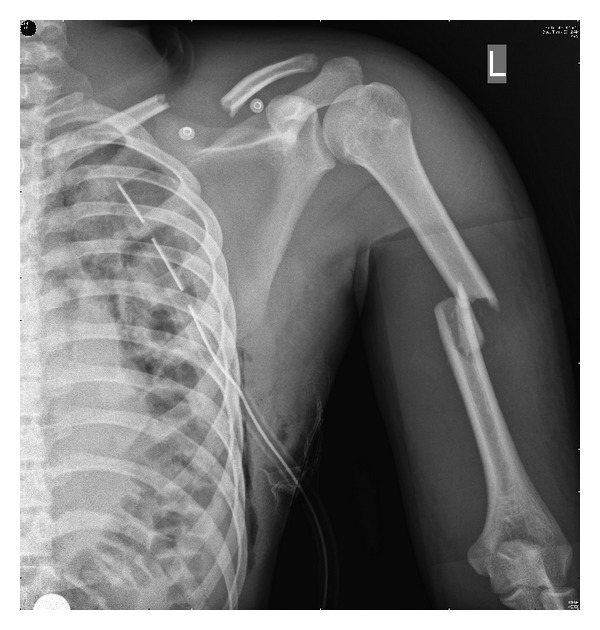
Plain radiograph showing a displaced fracture of clavicle and comminuted fracture shaft of humerus.
